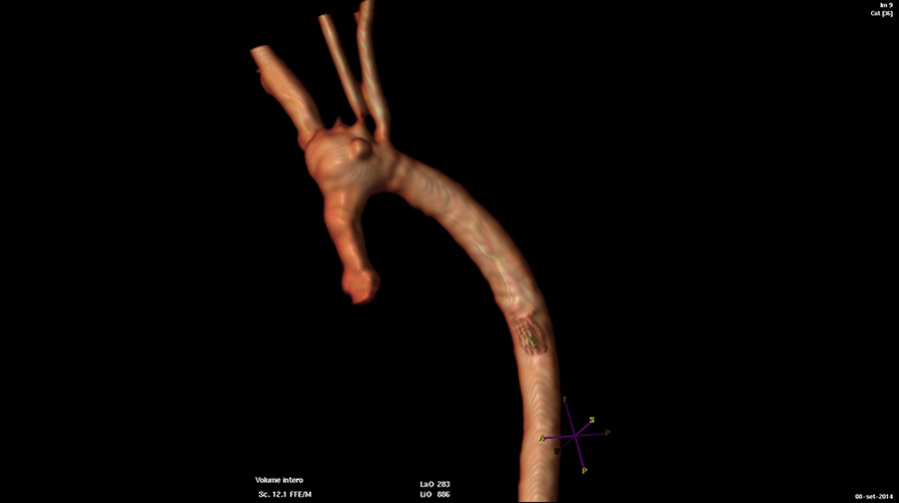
MRI 3D aortic arch reconstruction showing severe hypoplasia and multiple aneurysms of ascending aorta, extending till first brachiocephalic artery; sinotubular junction is also severely stenotic
